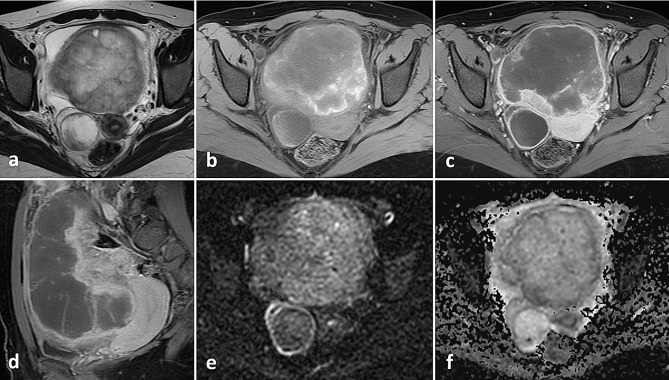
A left ovarian lesion of O-RADS MRI score 4, and a right ovarian lesion of O-RADS MRI score 3: (a) Axial T2-WI reveals a left-sided large multilobulated solid adnexal mass of intermediate signal intensity and irregular hypointense septa and a right ovarian cyst of mixed signal intensity. (b) Axial T1 fat-saturated image shows hypointensity of both lesions with few hyperintense areas denoting hemorrhagic changes. (c and d) Axial and sagittal T1 contrast-enhanced image; the left ovarian mass displays solid tissue and interlobular septal enhancement equal to the myometrium at 30–40 s, while the right ovarian cyst shows a smooth wall and septal enhancement with no detected enhancing solid tissue. (e) Axial DWI at b = 1000 revealed an intermediate signal of the left ovarian enhancing solid tissue and hypointensity at corresponding ADC image (f) denoting restricted diffusion pattern with an ADCmean value of 1.16 × 10 − 3 mm2 /s, the right ovarian cyst also revealed free diffusion pattern (apart from a small hemorrhagic area) with an ADCmean value of 2.02 × 10 − 3 mm2 /s. Pathology revealed left ovarian dysgerminoma and right ovarian benign hemorrhagic cyst
